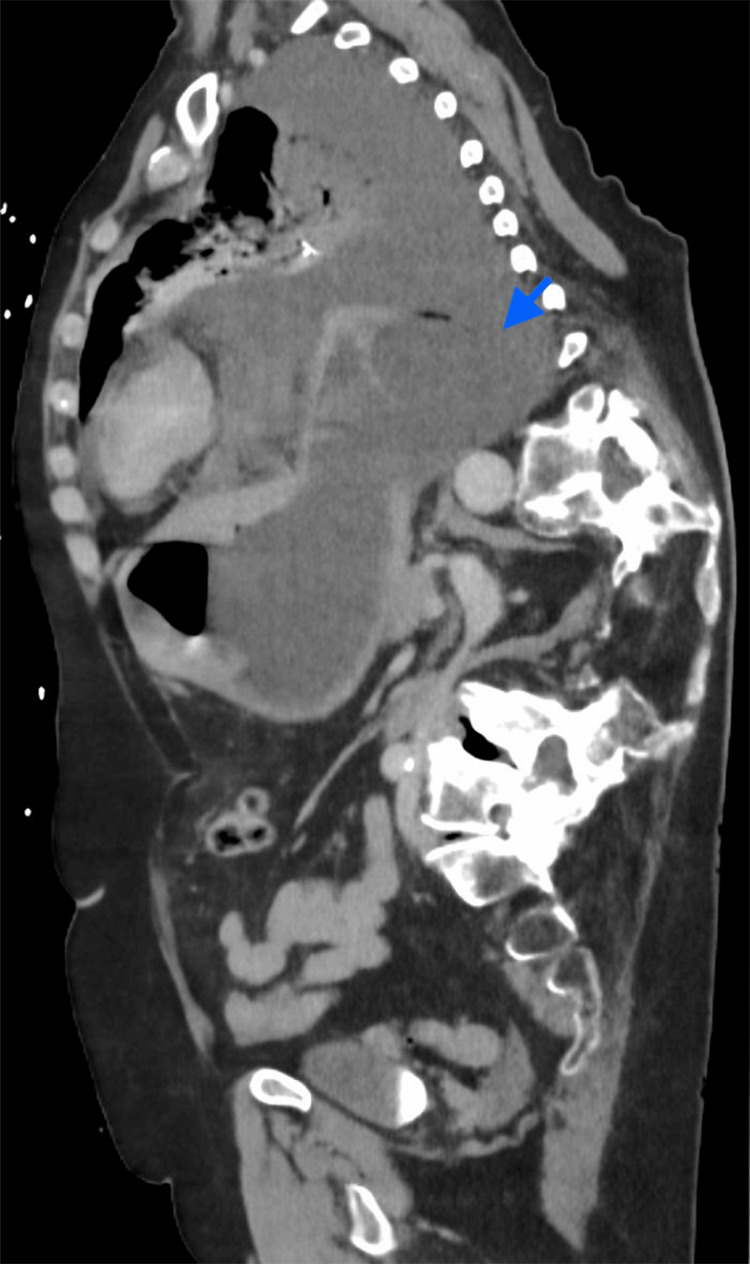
Sagittal contrast-enhanced CT image A large intrathoracic paraesophageal hernia with focal absence of enhancement of the posterior gastric wall (blue arrow), consistent with vascular compromise and transmural ischemia, is seen.
